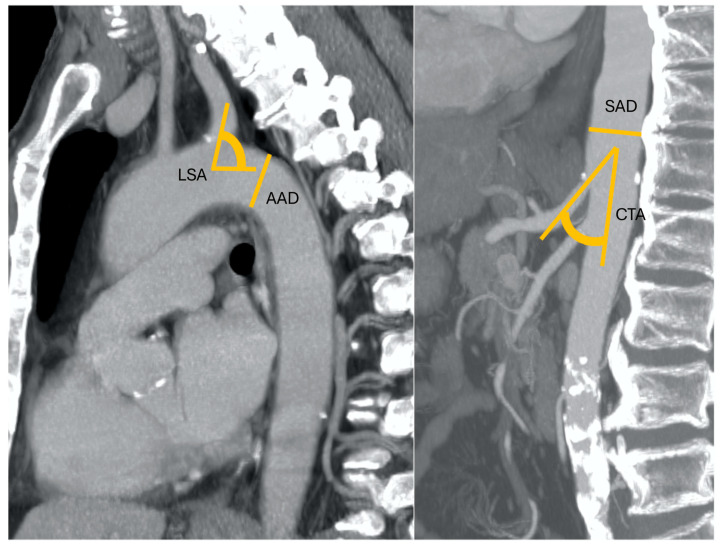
A 52-year-old patient with nodular HCC in the left liver lobe with a radial diameter (RD) of 3 mm (0 points), left subclavian artery angle (LSA) of 85° (0 points), an aortic arch angulation (AAA) type I (0 points) with a diameter (AAD) of 32 mm (1 point), suprarenal aorta diameter (SAD) of 23 mm (0 points), and celiac trunk angle (CTA) of 45° (0 points). Final RAD-access score: 0, transradial access preferred for values between 0 and 2.
